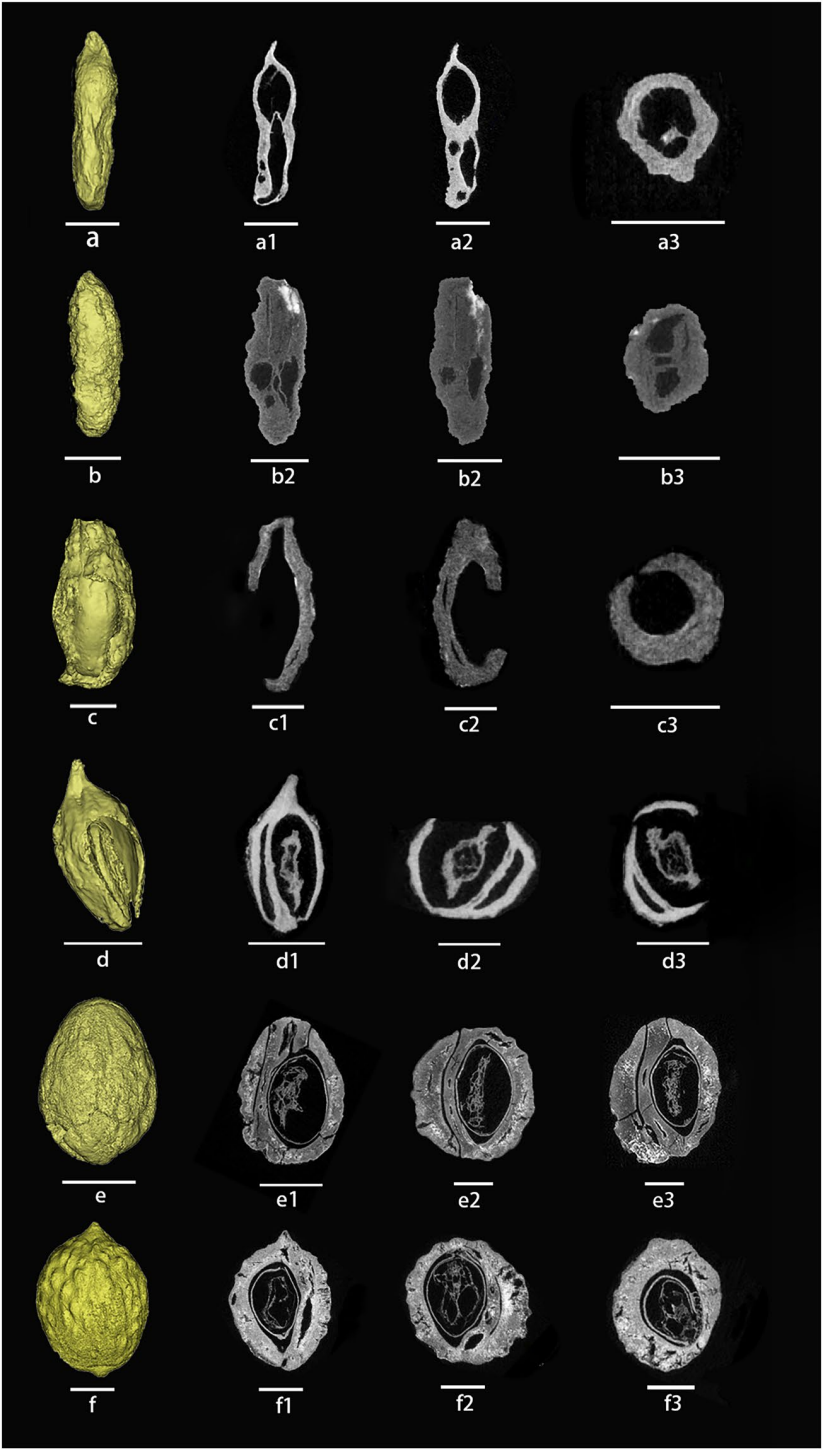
Three-dimensional structures were reconstructed using CT and the internal structure of jujube (a–d) and sour jujube (e, f) in different views of the sites. (a–c) Zhujia; (d) Wangzhuang; (e) Dalaidian; (f)Xiwusi. Scare bar = 2 mm.
